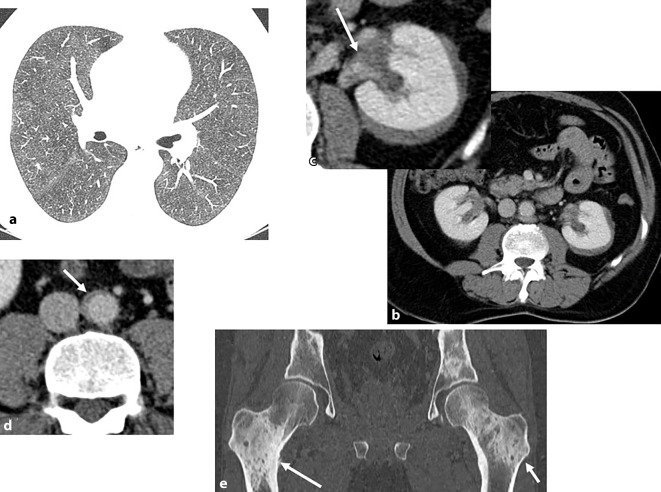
54-jähriger Patient mit bekannter Erdheim-Chester-Erkrankung. a Axiales Lungenfenster mit diskretem, jedoch ubiquitär vorherrschendem Ground-Glass. b Portalvenöse CT-Aufnahme des Abdomens mit charakteristischer renaler Beteiligung in Form einer ausgedehnten bilateralen, irregulären Infiltration des perirenalen Fettgewebes (Hairy-kidney-Zeichen) und fehlender kortikomedullärer Differenzierung (Featureless-kidney-Zeichen). Zusätzlich retroperitoneale Beteiligung mit periureteralem Weichteilplus um den rechten proximalen Ureter (Vergrößerungsaufnahme c , weißer *Pfeil*). d Periaortale Infiltration (Coated-aorta-Zeichen) durch Nicht-Langerhans-Zell-Histiozyten, was sich als periaortales, plaqueartiges, hypodenses Weichteilplus um die infrarenale Aorta darstellt (*weißer*
